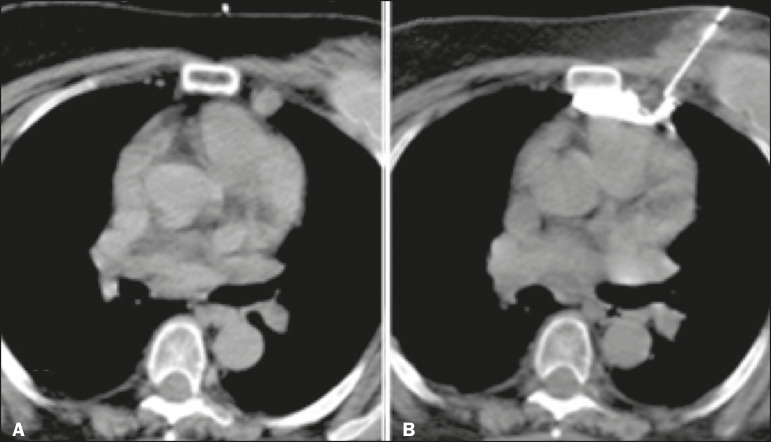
CT-guided percutaneous biopsy of a lesion in the right cardiophrenic space, adjacent to the liver. A: Coaxial needle positioned adjacent to the lesion. B: Hydrodissection with lidocaine gel and iodinated contrast after transfixation of the lesion with the coaxial needle.
